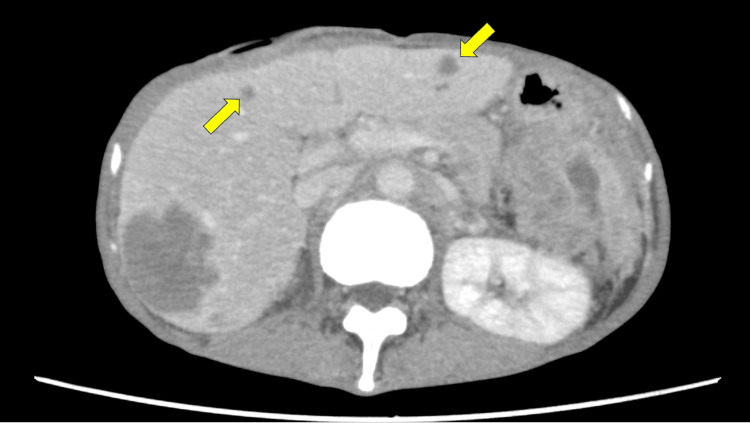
Abdominal CT after FOLFOX6 plus bevacizumab therapy. The scan shows the appearance of new liver metastases (arrow).
